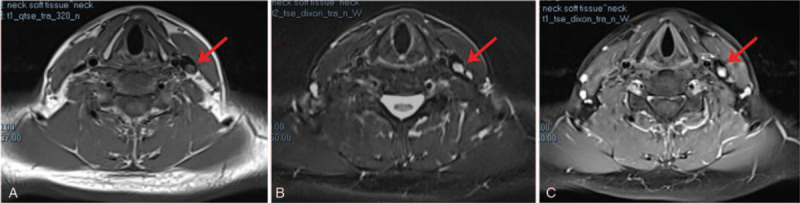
Contrast-enhanced magnetic resonance imaging (MRI) images: (A) The neoplasm is isointense on T1-weighted imaging; (B) The neoplasm is hyperintense on T2-weighted imaging; (C) Enhanced MRI image on T1-weighted imaging. The red arrows mark the neoplasm.
